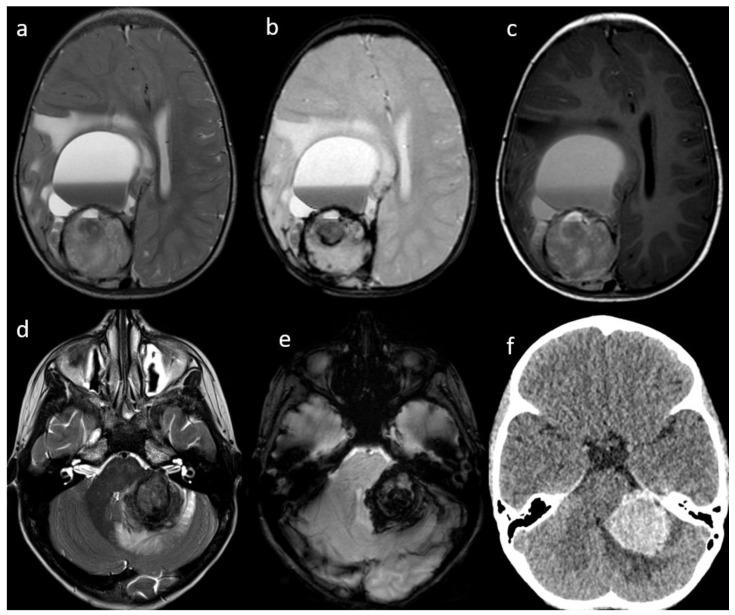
Patient #1 (a–c) and Patient #3 (c–e) imaging features. Axial T2w images of Patient #1 (a) and Patient #3 (d) shows a single bulky parenchymal lesion associated with mild surrounding vasogenic edema. Susceptibility-weighted imaging sequences in Patient #1 (b) and Patient #3 (e) show blooming within each lesion consistent with hemorrhage. Axial T1w image of Patient #1 (c) shows a solid-cystic appearance of the lesion with multiloculated blood-fluid levels in the most anterior portion and a solid hemorrhagic posterior component. Axial non contrast brain computed tomography (CT) of Patient #3 (f) shows lesion hyperdensity consistent with hemorrhage.
